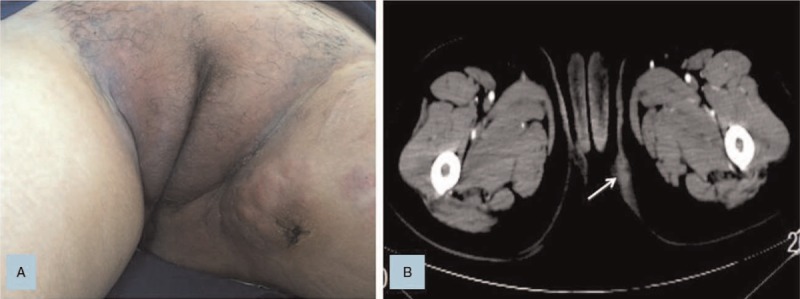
A. Nodules of the vulva were initially found. B. CT scan image for the tumor of the left vulva.
